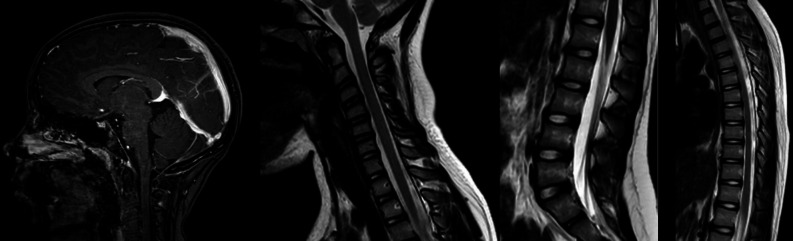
a-d Pre-procedure imaging: Brain MRI (a) and sagittal T2-weighted MRI of the cervical (b), lumbar (c), and thoracic (d) spine with no signs suggesting intracranial hypotension
